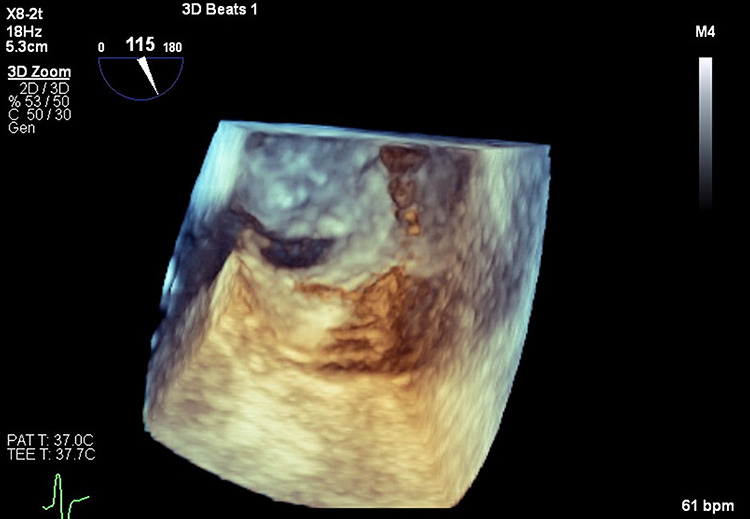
Three-dimensional transesophageal echocardiography with PFO in interatrial septum.
